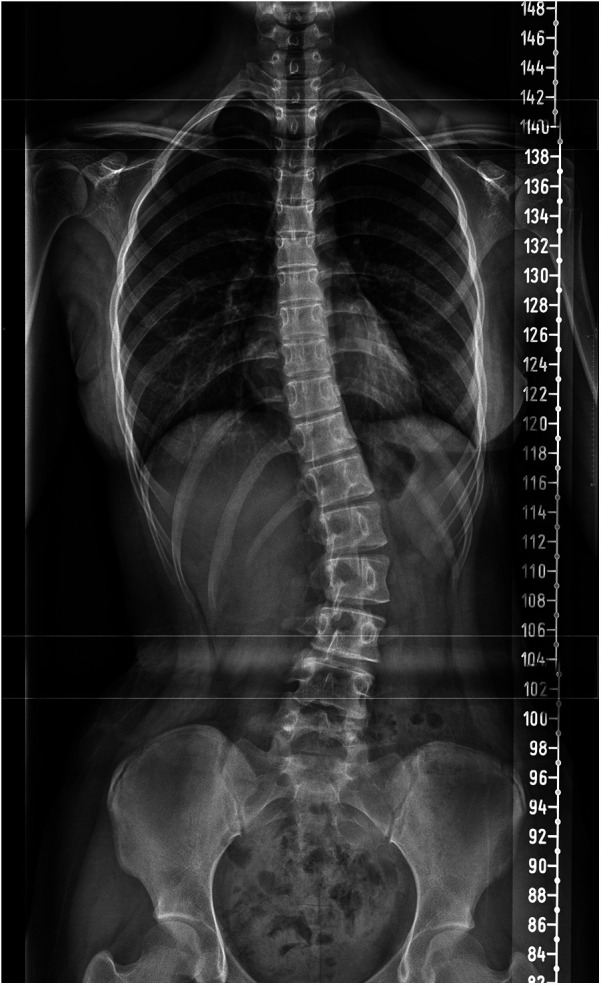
Representative spinal x-ray image of a 16-year-old girl diagnosed with adolescent scoliosis.
